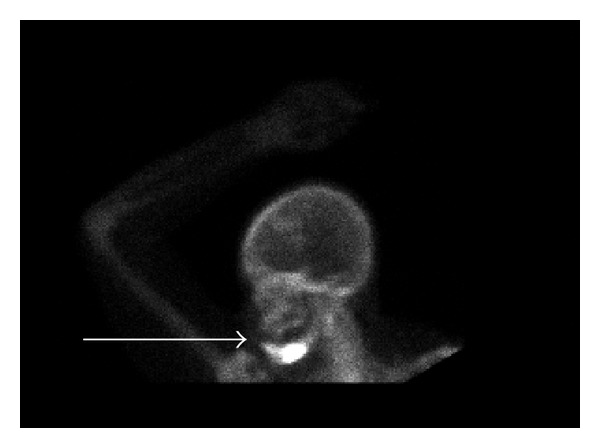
Technetium 99m Bone scintigraphy. Bone scan showing increased radioactive uptake in the body of the mandible (arrow) thought to be metastatic disease.
